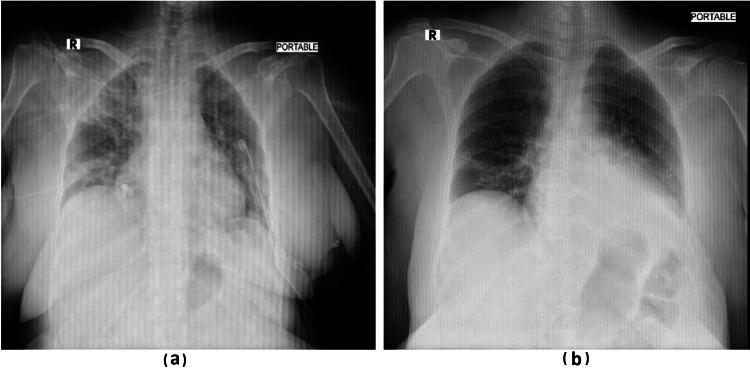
Postoperative chest x-ray showed no complications, and after the third postoperative day, the chest tube was removed. (a) Chest x-ray before chest tube removal and (b) chest x-ray after chest tube removal.
